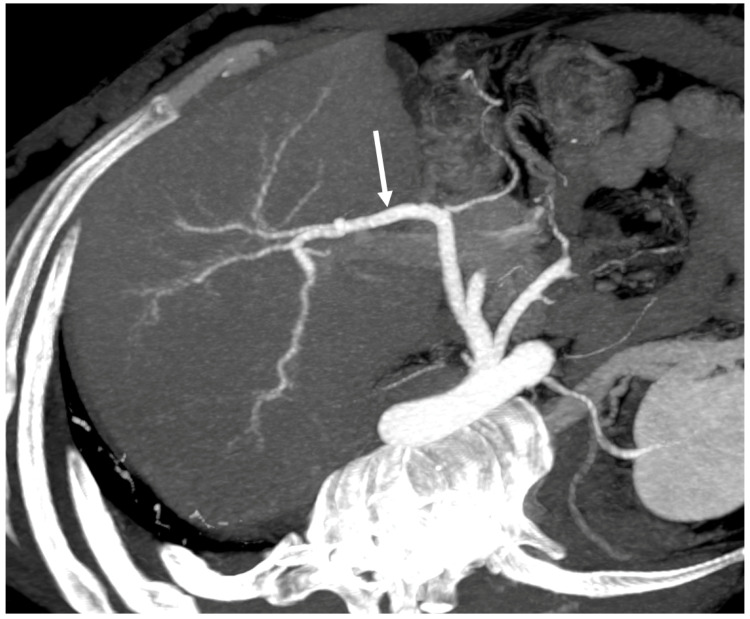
Maximum intensity projection CT reconstruction exhibits the enlargement of the hepatic artery (arrow).
